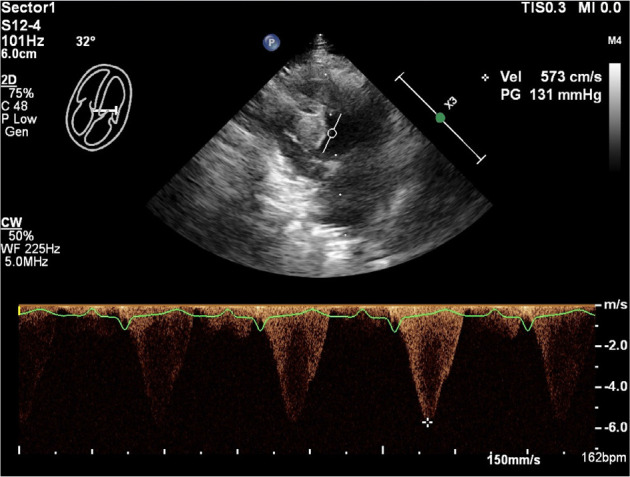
Continuous-wave Doppler echocardiographic images of the left ventricular outflow tract Peak velocity of 5.73 m/s and a pressure gradient of 131 mmHg were measured, consistent with severe dynamic left ventricular outflow tract obstruction
